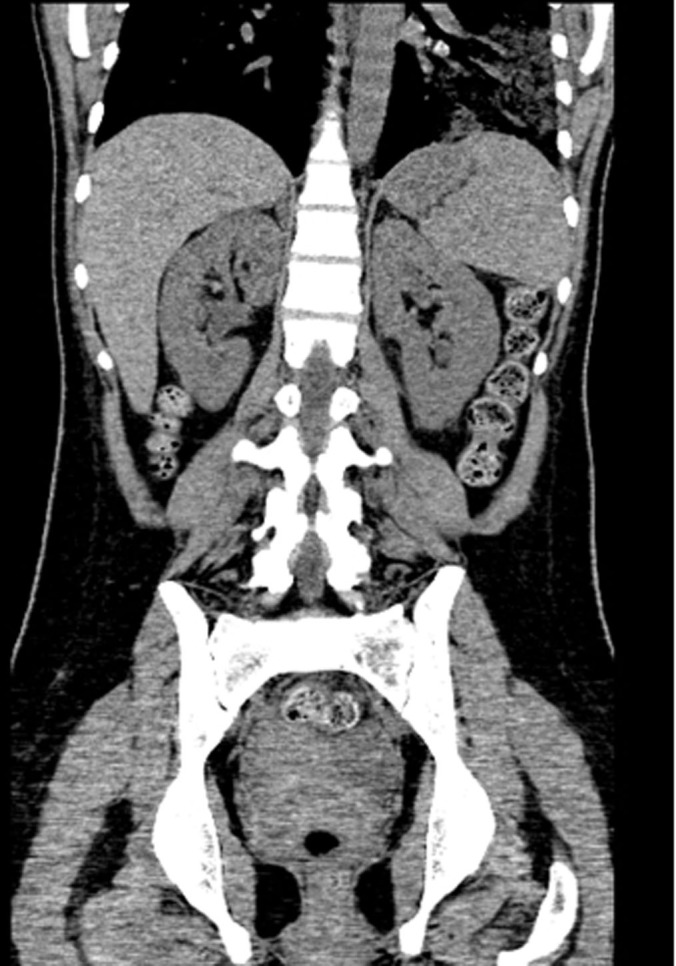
Coronal view of abdominal computed tomogram without contrast showing air in the collecting system of both kidneys.
